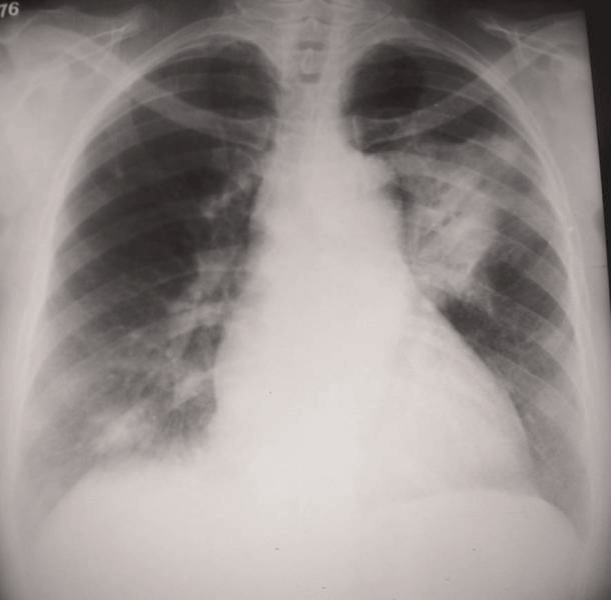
Chest X-ray shows a large consolidation with air bronchograms in the left lung, and a small area of consolidation in the lower region of the right lung.
